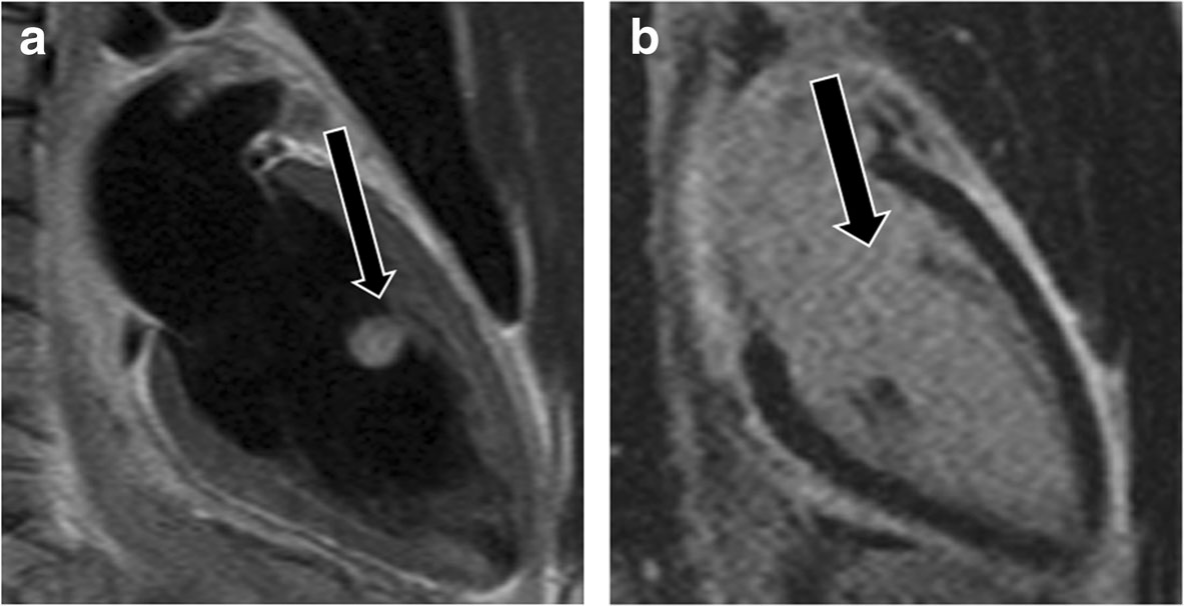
Myxoma. a Two-chamber T2-weighted dark blood image shows a hyperintense mass involving the anterolateral papillary muscle (arrow). b The mass enhances after contrast-enhancement (arrow) making it isointense to the blood pool. This was proven to be a myxoma
